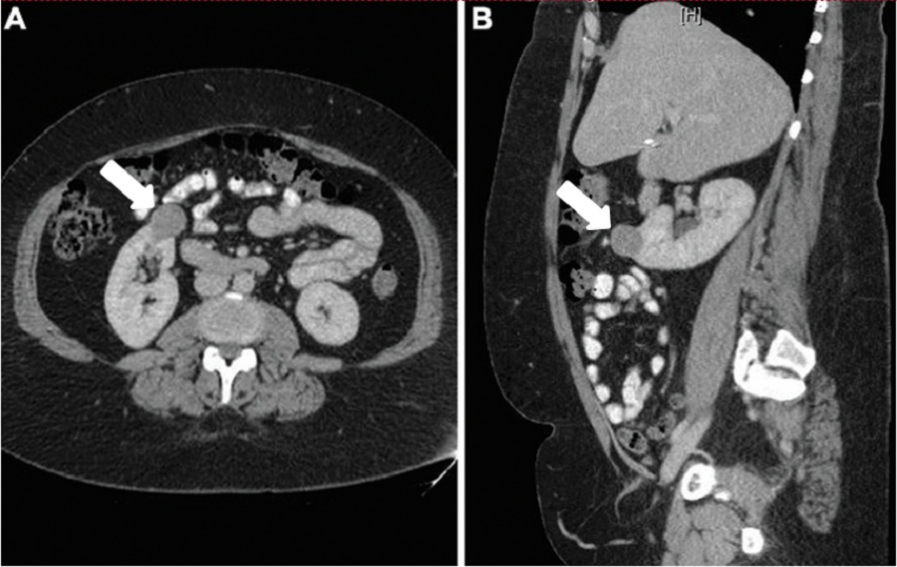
Representative contrast computed tomographic images of the renal mass A) axial and B) sagittal planes
